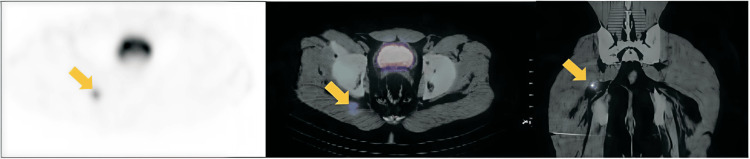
SPECT/CT with 99mTc-HYNIC-TOC Overexpression of somatostatin receptors (Krenning score 3–4) demonstrated by increased radiotracer uptake in a 1.4 × 1.1 cm nodular lesion located between the right gluteus maximus and inferior gemellus muscles (yellow arrows). SPECT: Single-photon emission computed tomography; 99mTc-HYNIC-TOC: Technetium-99m-hydrazinonicotinyl-Tyr^3^-octreotide.
